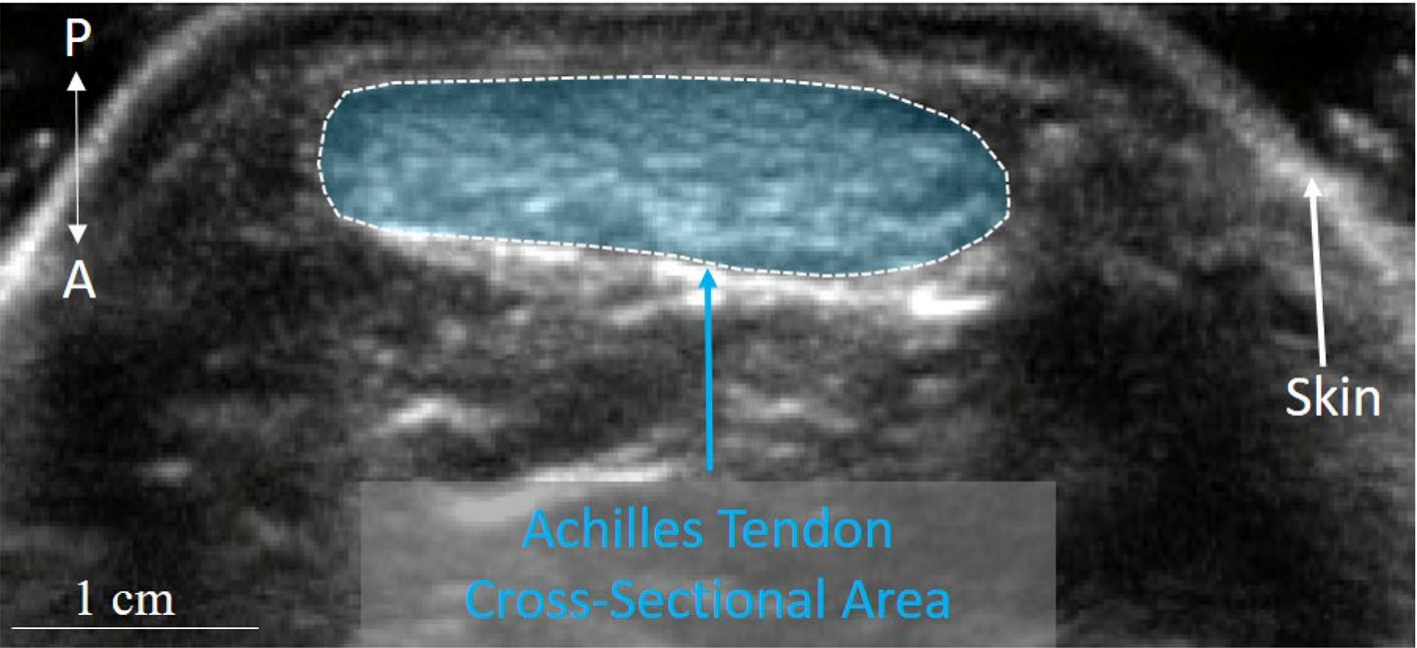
The cross-sectional area of the Achilles tendon measured using ultrasound. Example of the cross-sectional area of the Achilles tendon measured at the level of the malleoli using B-mode ultrasound. The area of the tendon outlined by the dashed white line and shaded in blue was calculated using ImageJ. A is anterior and P is posterior. The hyperechoic region anterior to the shaded ellipse around the tendon is Kager’s fat pad.
